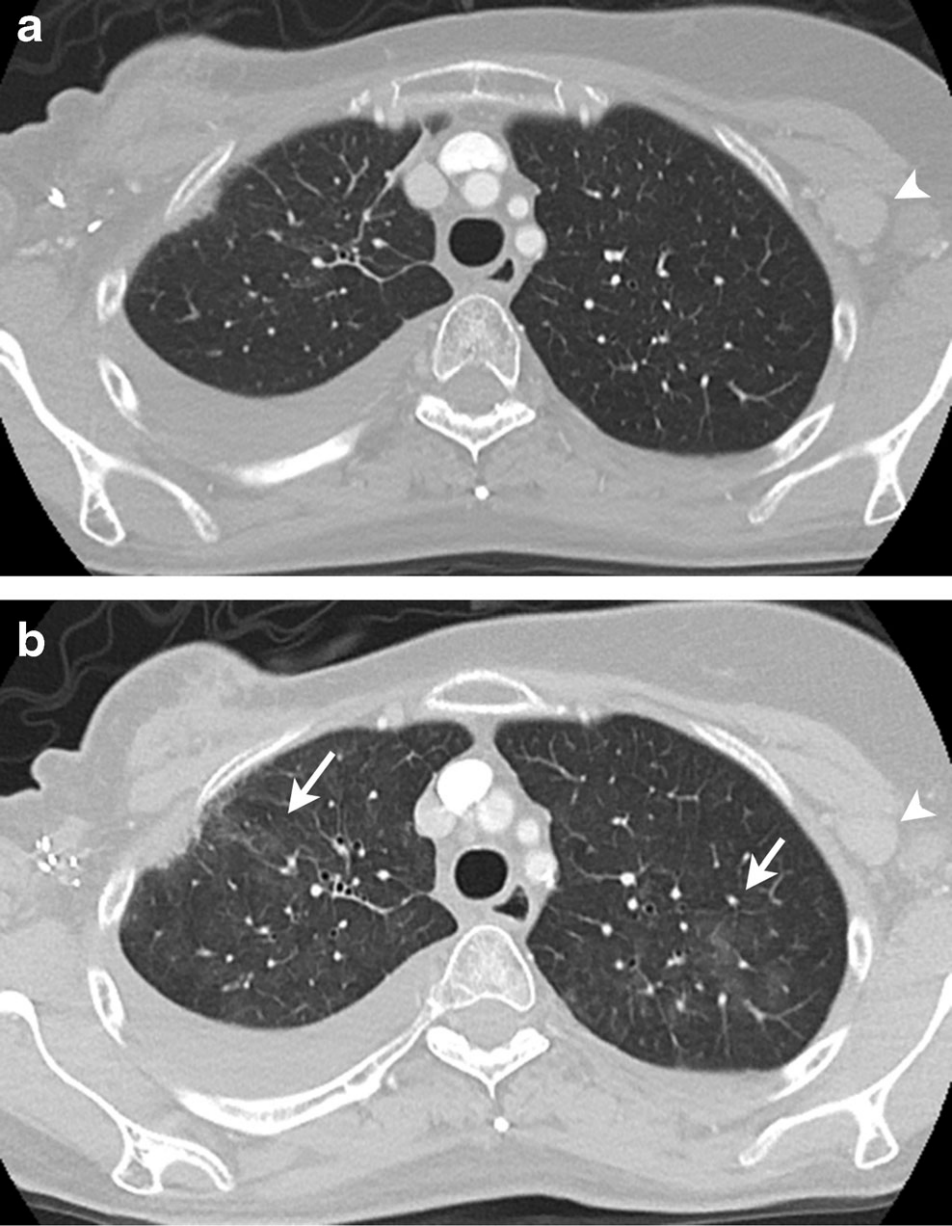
69-year-old woman with metastatic hormone receptor-positive, HER2/neu-positive breast cancer being treated with paclitaxel. Axial contrast-enhanced lung window CT image before (a) and after (b) treatment with paclitaxel shows interval appearance of patchy ground-glass opacities in upper lobes of lungs (*arrow* in b) suggestive of drug-associated pneumonitis. Also note the decreased size of left axillary lymph node (*arrowheads* in a and b) suggestive of treatment response
